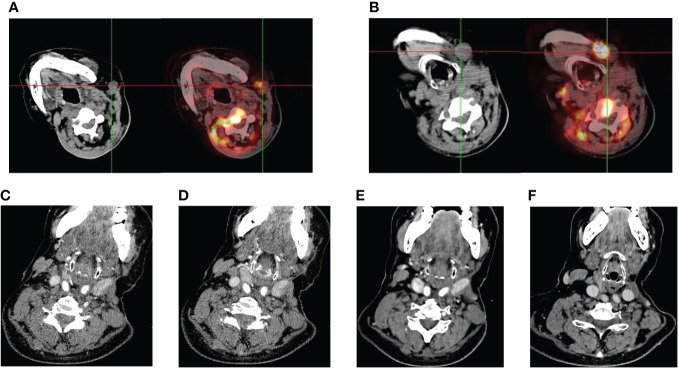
Imaging performance. (A, B) Eight months after the second surgery, the patient’s PET/CT examination suggested tumor recurrence and metastasis with multiple lymph node metastases in the left side of the neck. (C–F) continuous shrinkage of the tumor lesion and metastatic lymph nodes following sintilimab combined with anlotinib treatment in October 2023, December 2023, respectively.
